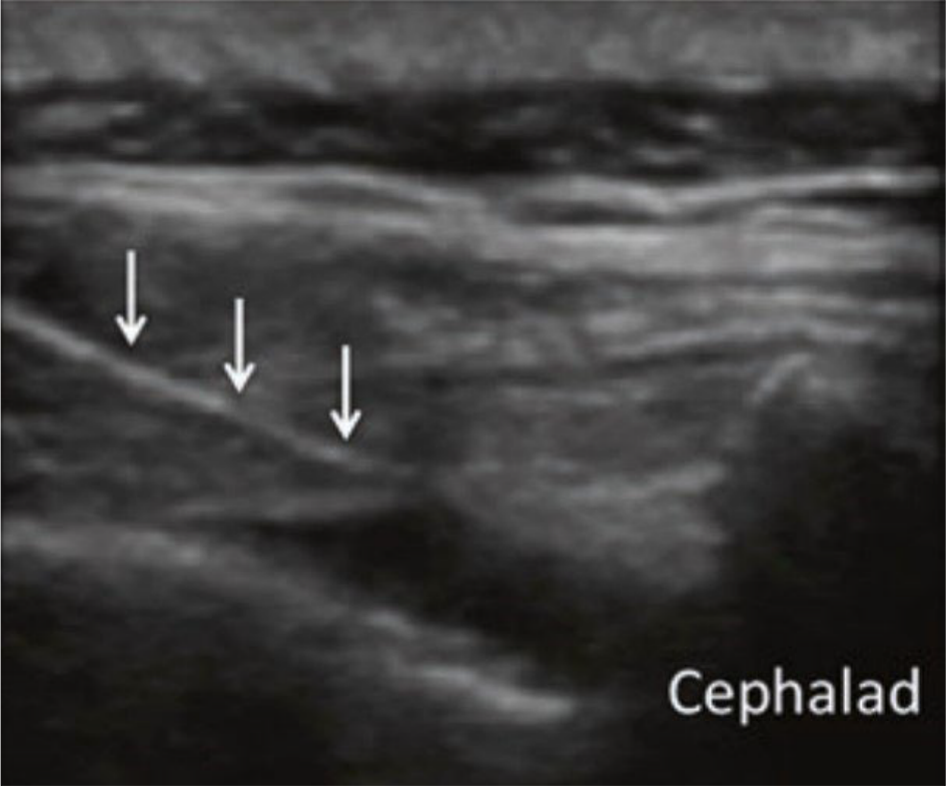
The caudal ESI ultrasound view
